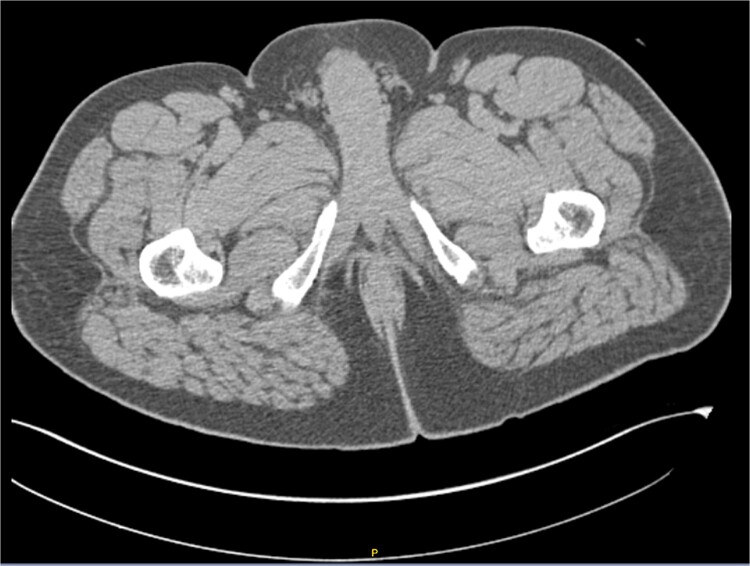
Computed tomography (CT) scan with intravenous contrast (IV). Hepatic cystic lesions, with diameters of up to 5 mm, which did not enhance with contrast. Additionally, it was observed that the perianal region showed no fat stranding or increased volume, and no lymphadenopathy was detected.
